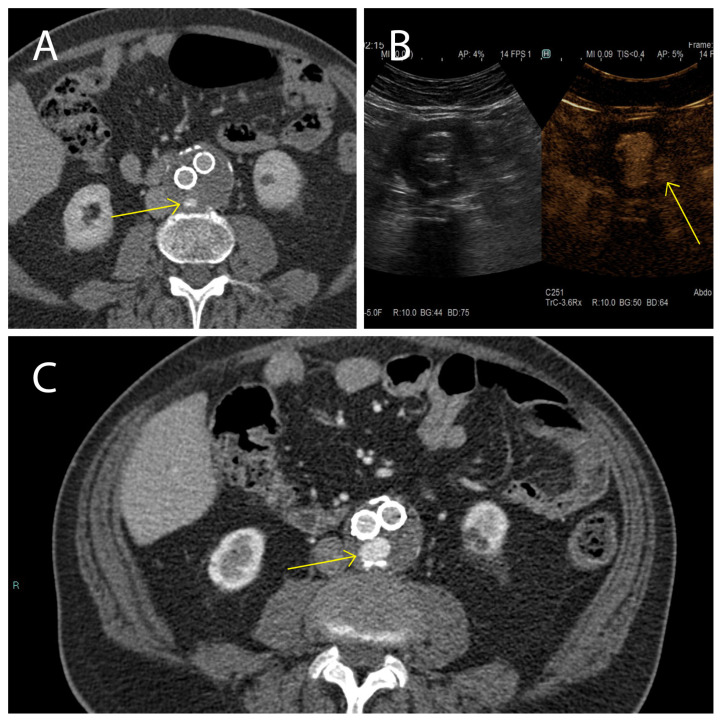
Presence and persistence of an endoleak. In these images a type II (yellow arrow) was evidenced using CTA 1 month after EVAR intervention, (A) monitored during the follow-up after 6 months using CEUS (B), and again using CTA 12 months after EVAR implantation (C).
